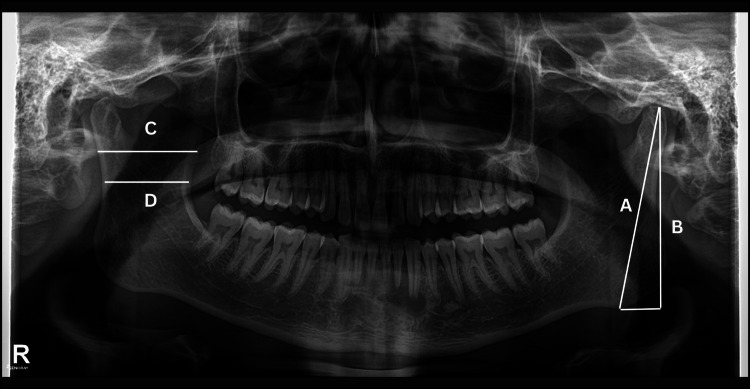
OPG demonstrating the linear measurements of mandibular parameters using GIMP GIMP, GNU Image Manipulation Program; OPG, Orthopantomogram
